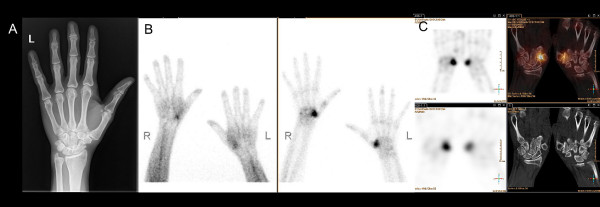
A 48-year-old female with symptomatic bilateral CMC-1 osteoarthritis. The patient had a history of trapezectomy on the left hand 3 years ago. (A) Plain radiographs showed moderate CMC-1 osteoarthritis on the left side. (B) Intense uptake in the right CMC-1 joint and moderate uptake in the left CMC-1 joint on early-phase imaging (left), intense radioisotope uptake in both CMC-1 joints on late-phase imaging (right). (C) SPECT/CT confirmed activated bilateral rhizarthritis/osteoarthritis with neoarticulation of the MC-1 and trapezoid bone on the right side. Patient received re-arthroplasty (top row: 3D-SPECT and fusion SPECT/CT, bottom row: planar SPECT and CT-alone).
